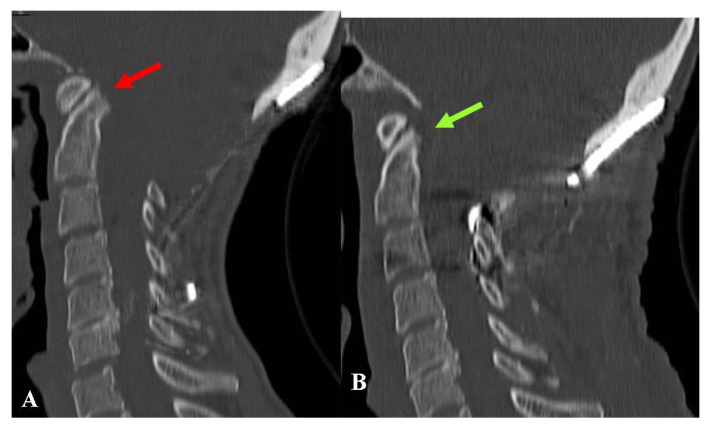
This 43-year-old woman had undergone osteodural decompression and craniocervical fixation. Six months later, she was referred to us due to progressively worsening swallowing disturbances, severe weight loss, asthenia, and marked continuous occipital pain. (A) Preoperative CT-scan, sagittal reconstruction showing a relatively wide bone decompression and a basilar impression with a quite acute clivo-axial angle (red arrow). Under neurophysiologic and radiologic monitoring, the patient underwent the removal of the fixation device and cranial traction. The basilar impression could be reduced, which made the planned procedure of odontoidectomy unnecessary. New craniocervical fixation devices were placed to maintain the new position. (B) Follow-up CT-scan, sagittal reconstruction, obtained one year later, showing resolution of the basilar impression (green arrow). The swallowing problems almost completely went away, and the headache got better, but the asthenia stayed. The final CCOS score was 10.
